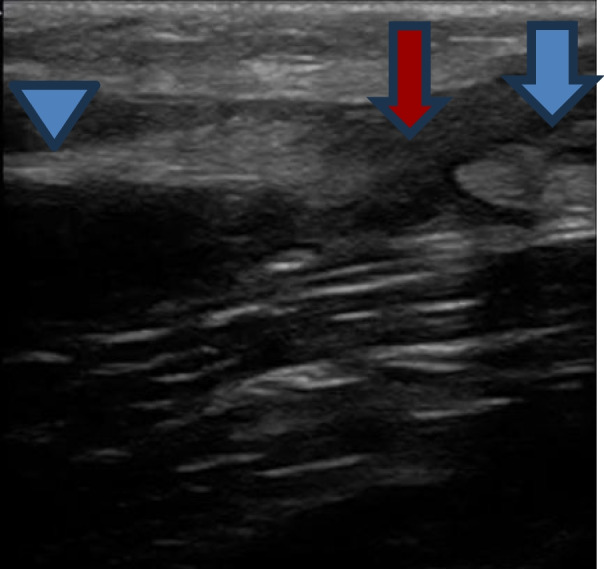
Sagittal sonographic view demonstrating a ruptured distal biceps tendon. Proximal end (blue arrow), distal end (arrowhead), and discontinuous area with surrounding hematoma (red arrow) were visualized
